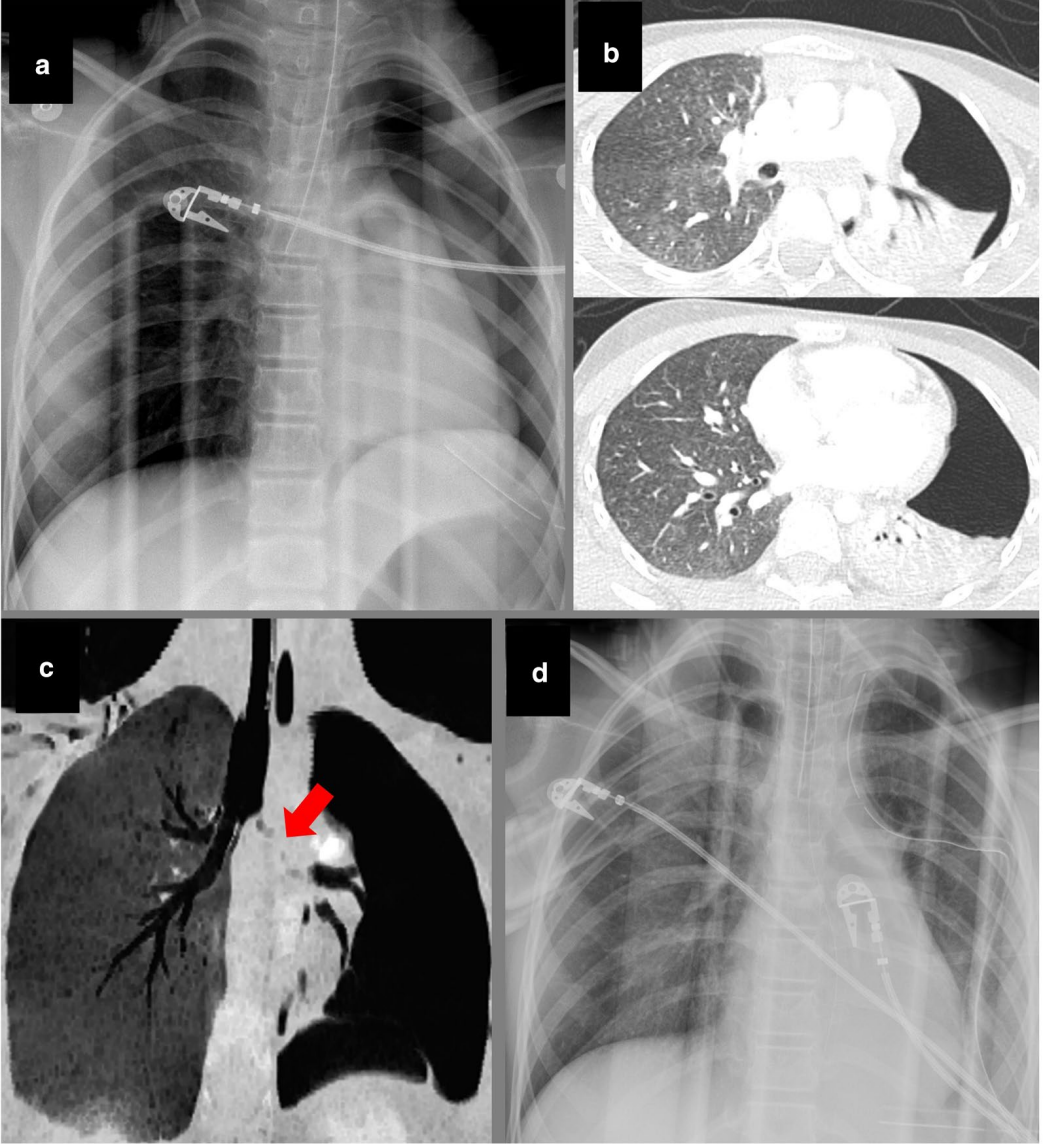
Faulty reasoning (type 2) error with anchoring and confirmation bias. A 10-year-old female patient was admitted to the ER after MVA. She had signs of severe head trauma and was intubated in the ER due to altered mental status. Following intubation, the absence of left lung ventilation was noticed, and a chest tube was placed in the left lung. Left-sided pneumothorax and misplaced intubation cannula extending to the bronchus intermedius were seen on the control chest radiograph (a). After stabilizing the vital signs, thorax and abdomen CTs were performed in the second hour of admission to assess the severity of the traumatic injury. Thorax CT showed “collapsed left lung with pneumothorax” (b, c) and “discontinuity of left main bronchus” (red arrow, c). The on-call radiologist evaluated those findings in favor of “bronchial rupture” and “fallen lung sign.” After making a preliminary report, the radiologist was called by a clinician, who informed him that patient’s intubation was traumatic and difficult, and asked him whether these findings could be secondary to “acute bronchial obstruction and subsequent collapse.” The radiologist rejected this possibility without thinking and stated that the findings he saw were compatible with bronchial rupture. Following the telephone call, the radiologist scanned the literature and read that bronchial rupture is usually seen with severe accompanying thoracic injuries and is associated with pneumomediastinum and pneumothorax. Although the radiologist knew that the patient did not have any other thoracic injury or pneumomediastinum, he sought exceptional cases that supported his pre-diagnosis, and he stuck with his first decision. The patient’s poor general condition did not allow surgical intervention or bronchoscopy. She was followed up with chest tube drainage and bronchial aspiration. On the second day after admission, portable chest radiography showed that the left lung ventilation was markedly normalized (d). When the findings were evaluated retrospectively, it was understood that bronchial rupture was unlikely, and the diagnosis was compatible with “acute lung collapse and pneumothorax ex vacuo secondary to the traumatic intubation.” Thus, the radiologist, under the influence of anchoring confirmation bias, had falsely attributed the findings he detected in favor of a false pathology
